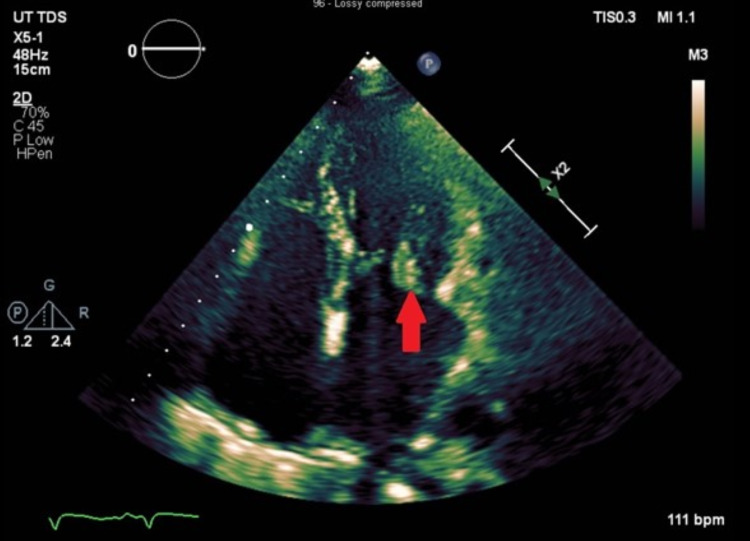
Mitral valve vegetation on the posterior leaflet seen in the apical four-chamber view transthoracic echocardiogram
